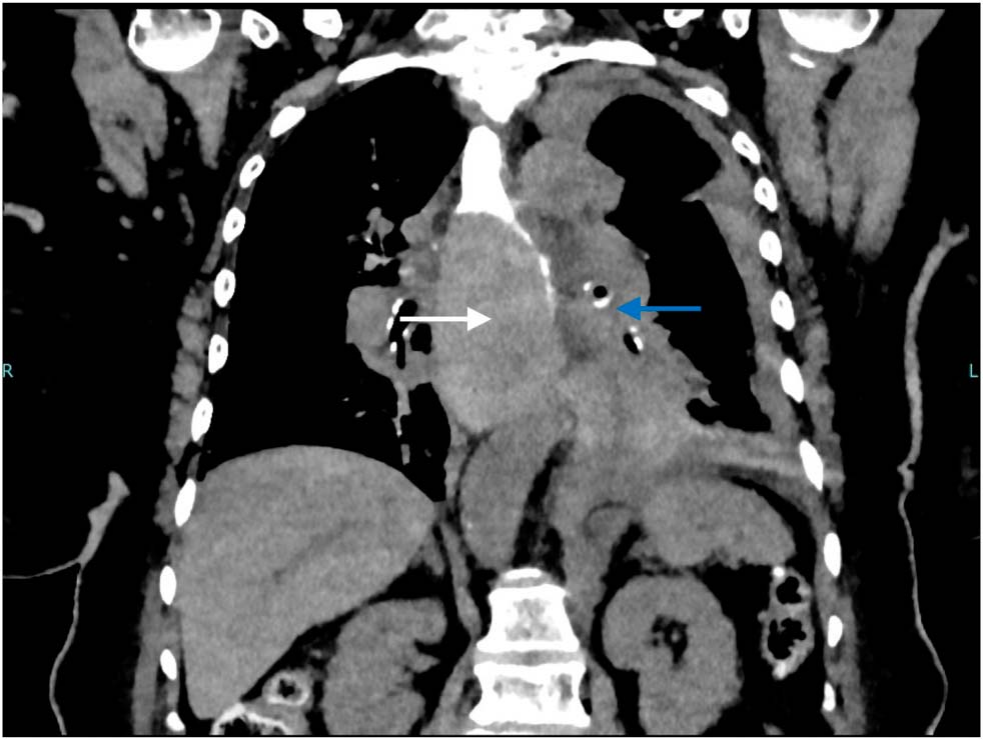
Contrast computed tomography scan showing esophageal hematoma (white arrow) with associated hemorrhagic effusion (blue arrow).
